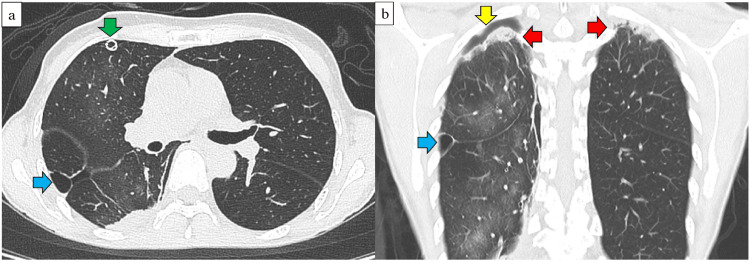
Computed tomography at the initial examination a) After insertion of right chest tube (horizontal section). A cyst (blue arrow) is observed between the right middle and lower lobes; b) After insertion of the right chest tube (coronal section). Infiltrative shadows (red arrows) are observed in both lung apices. An air space (yellow arrow) is observed in the right lung apex. A cyst (blue arrow) is observed between the right middle and lower lobes.
